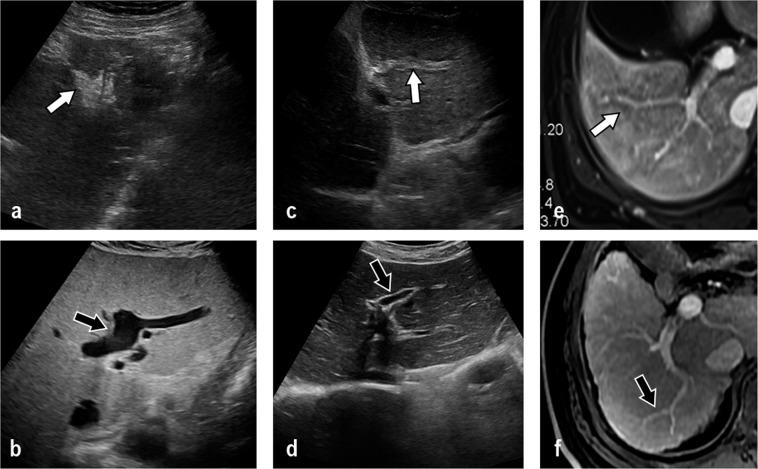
Images show the changes in the sagittal portion and other branches of the portal vein. (a) The ultrasound image shows the thickened vascular wall and nearly occluded lumen (white arrow) in the sagittal portion of the portal vein in an idiopathic portal hypertension (IPH) patient. (b) The ultrasound image shows the thin vascular wall (black arrow) and dilated lumen of the sagittal portion of the portal vein in a cirrhosis patient. (c) The ultrasound image shows the thickened vascular wall and nearly occluded lumen (white arrow) in the S3 portion of the portal vein in an IPH patient. (d) The ultrasound image shows the thin vascular wall (black arrow) and dilated lumen of the S3 portion of the portal vein in a cirrhosis patient. (e) The contrast-enhanced MR T1WI (TR 4.8, TE 2.4) image shows the thickened and rough vascular wall (white arrow) in an IPH patient. (f) The contrast-enhanced MR image shows no significant change in the S3 portal vein vascular wall in a cirrhosis patient.
